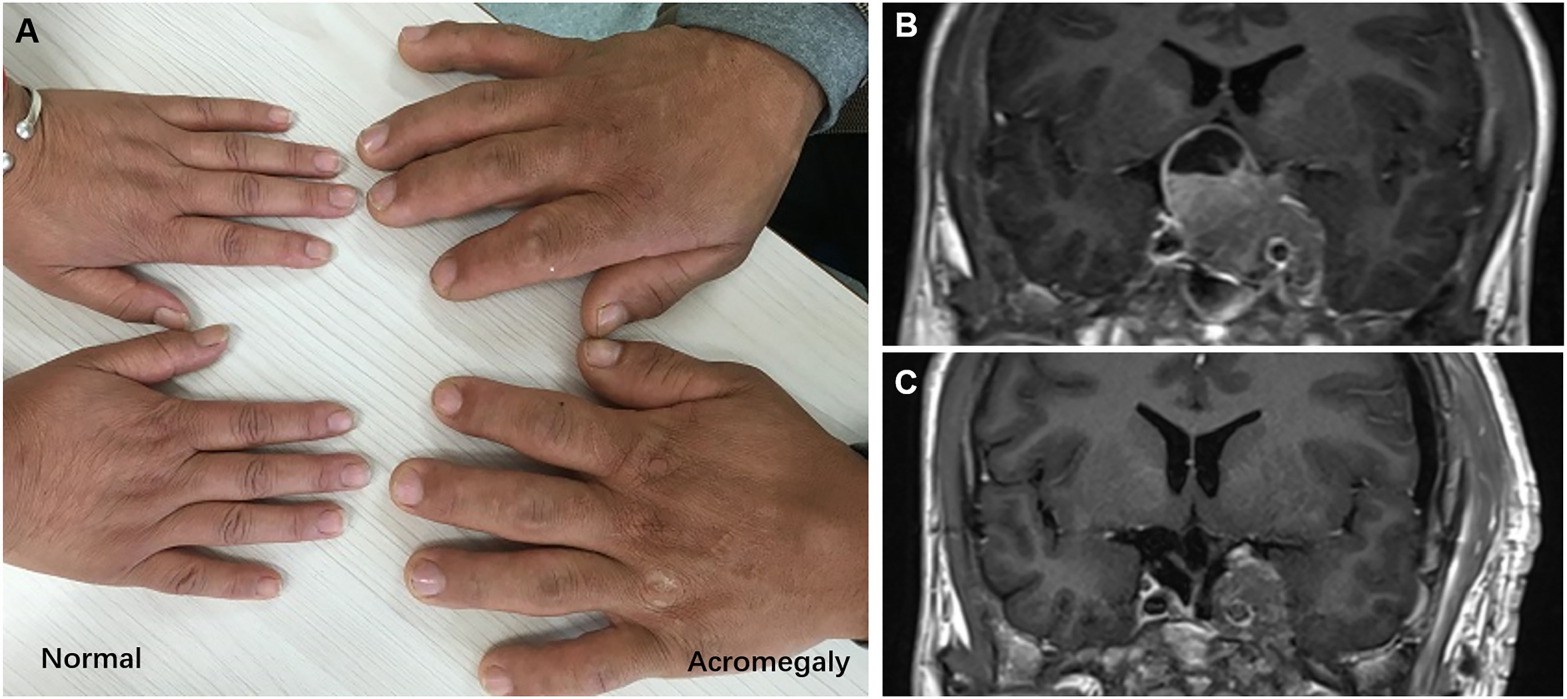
(A) The hands of normal and acromegaly people. (B) Preoperative MRI, 44mm× 27mm×37mm in size, with left cavernous sinus involvement, and internal carotid artery encased. (C) Postoperative MRI, show the residual tumor encircling the left internal carotid artery.
